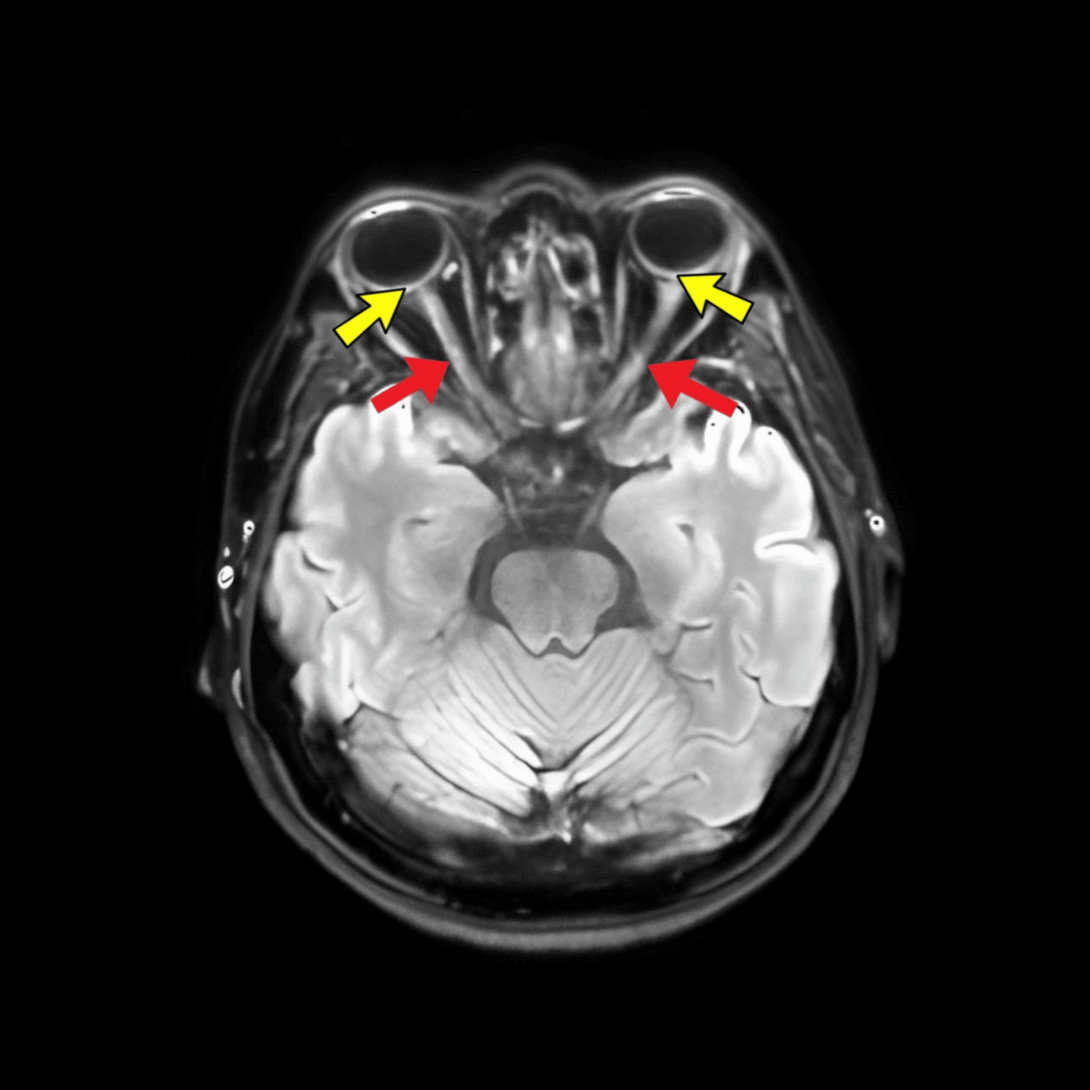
MRI brain and orbit showing enhancement of both optic nerve (red arrows) with posterior scleritis (yellow arrows)
